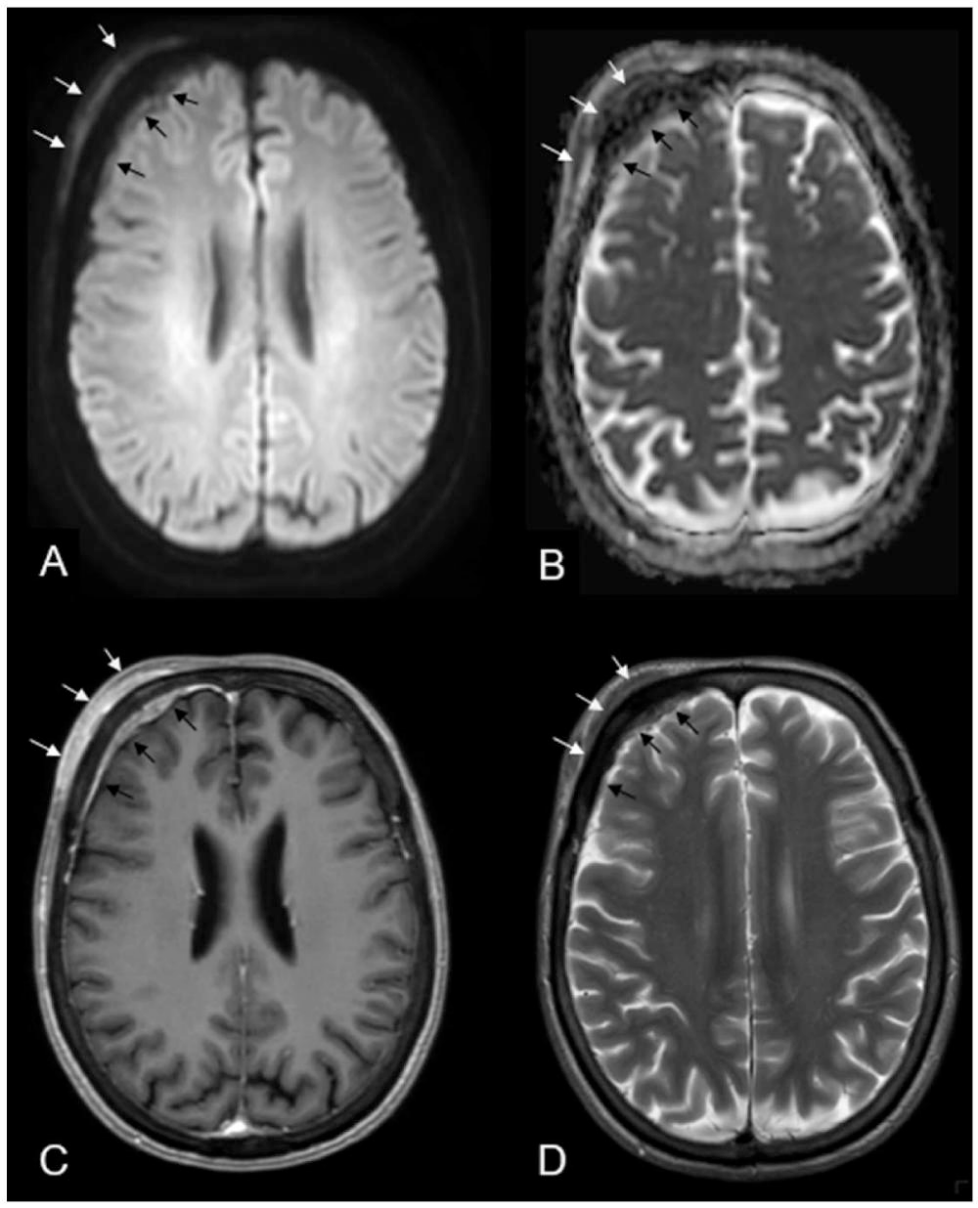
Extra- (white arrows A–D) and intracranial (black arrows A–D) transosseous lesion with diffusion restriction; particularly in the extracranial component, along with very low signal intensity on T2-weighted images and avid, nearly homogeneous contrast enhancement of the extra- and intracranial tumour components.
